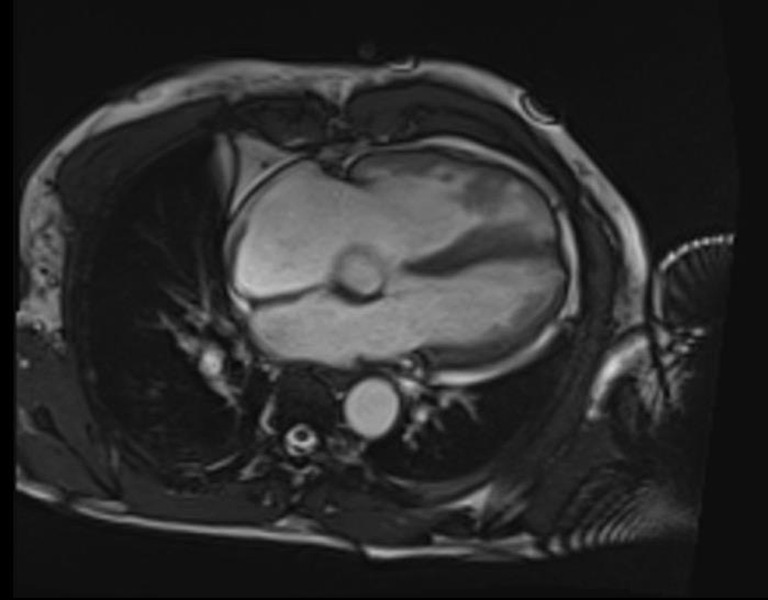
Four-chamber steady-state free procession end-diastolic bright blood cardiac MRI image showing mildly dilated RV and irregular RV wall
